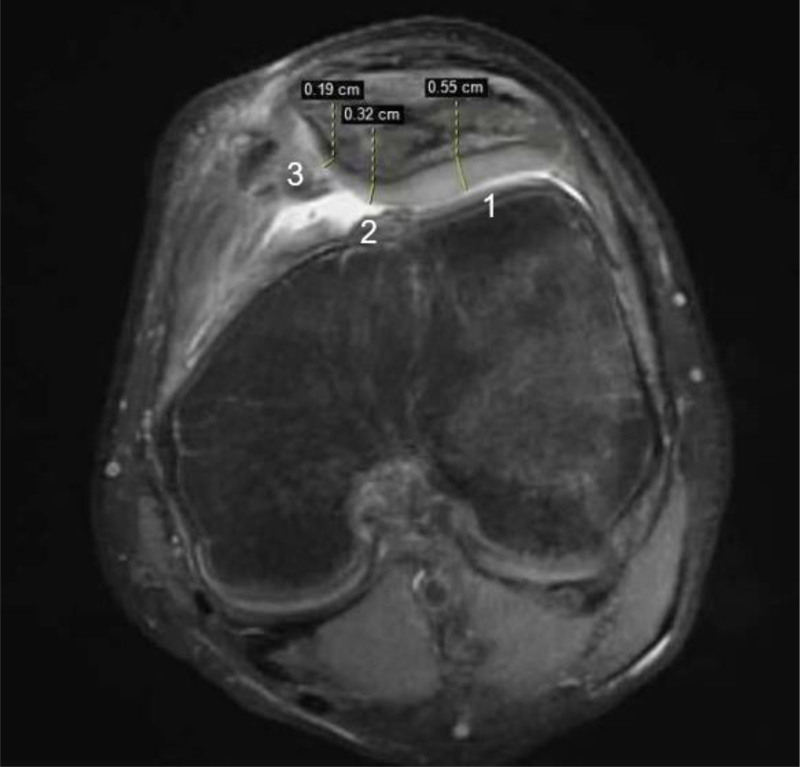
MRI cross-section with reference points.
